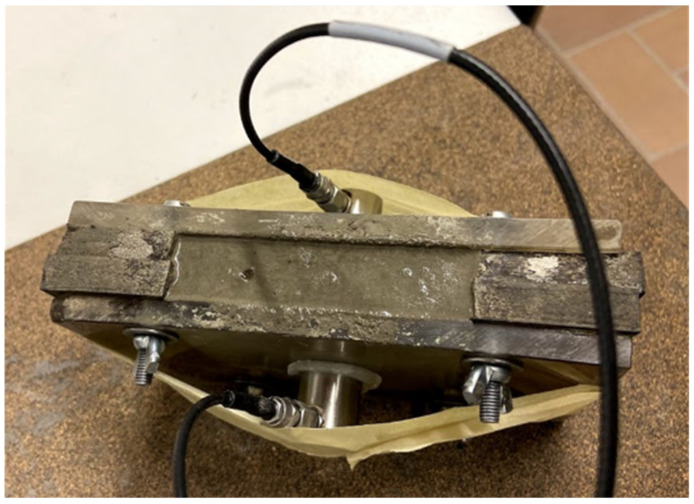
Ultrasound set-up showing the mortar sample in between both sensors.
